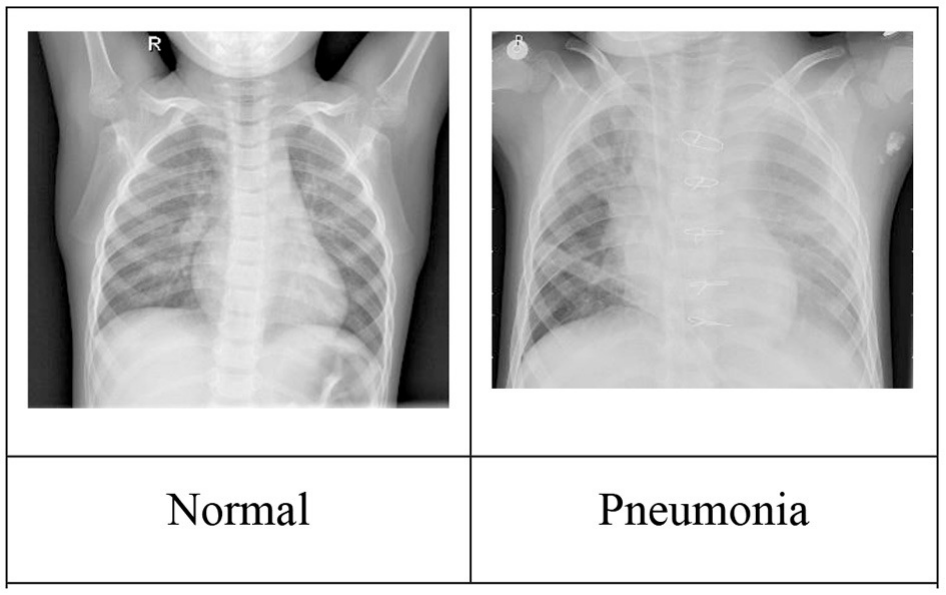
Sample chest X-ray images. Image source: Kermany (2018).
